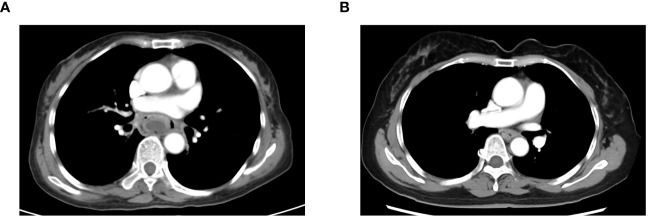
Representative contrast-enhanced chest CT images of the ESC and ESCC patients. (A) A CT image of a 57-year-old woman with pathological stage II ESC on the middle thoracic location showed that the tumor presented as a large lump growing into the lumen with an unclear boundary, and the peripheral lesion had a moderate enhancement. (B) A CT image of a 63-year-old man with pathological stage III ESCC on the middle thoracic location showed that the tumor presented as a thickening of the esophagus wall with an even enhancement. Both CT images were taken at the arterial phase. ESC, esophageal sarcomatoid carcinoma; ESCC, esophageal squamous cell carcinoma.
